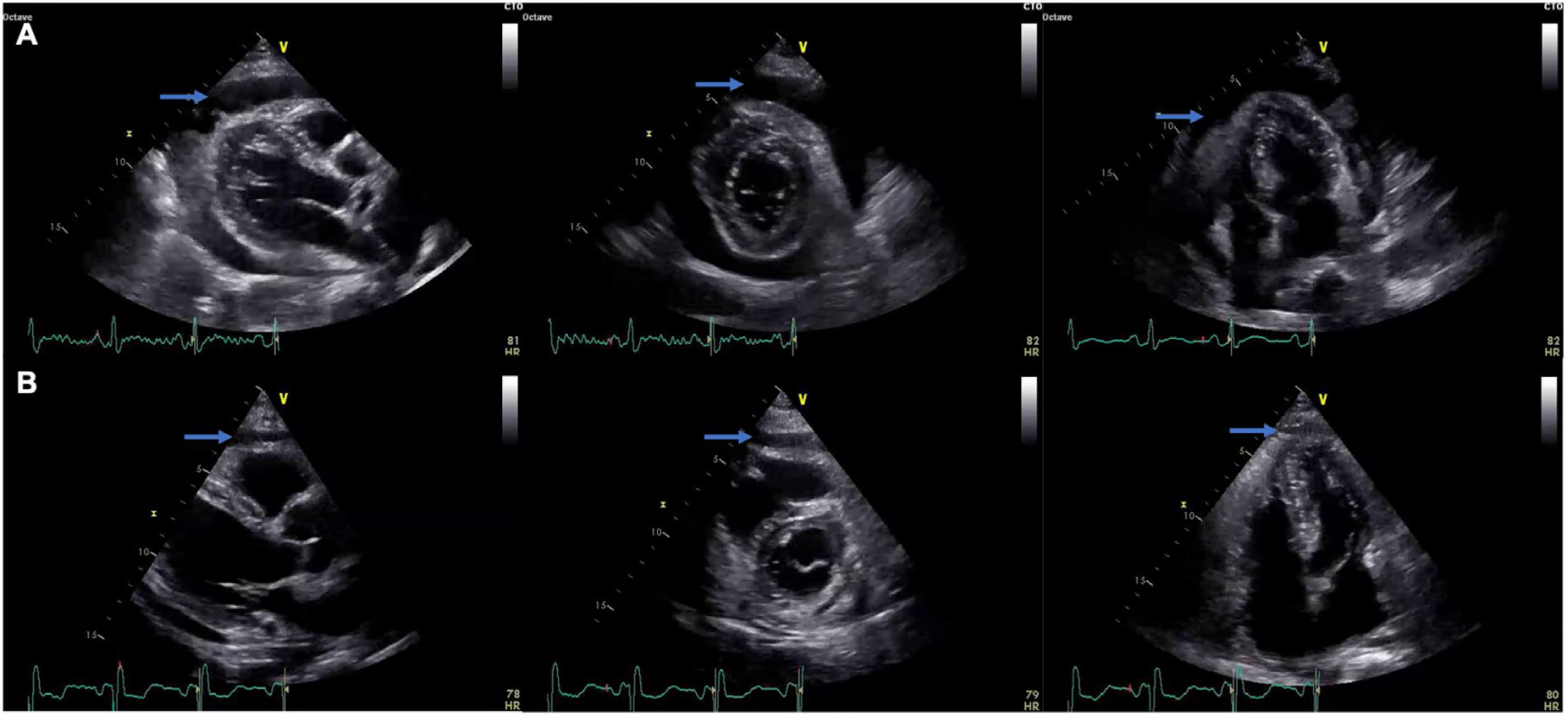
Transthoracic Echocardiograph. A) At the cardiac intensive care unit there were signs of hemodynamic effects with a collapse of the right ventricle in diastole and collapse of the right atrium in systole. The pericardial effusion increased to about 2 cm. There was a high-echogenic structure suspicious for fibrin deposition. B) The pericardiocentesis resolved the hemodynamic instability. The pericardial effusion was culture negative for mycobacteria. Negative PCR for M. tuberculosis-complex-DNA but positive sequencing (16S) for Neisseria meningitidis DNA. Blue arrow pointing towards pericardial effusion.
